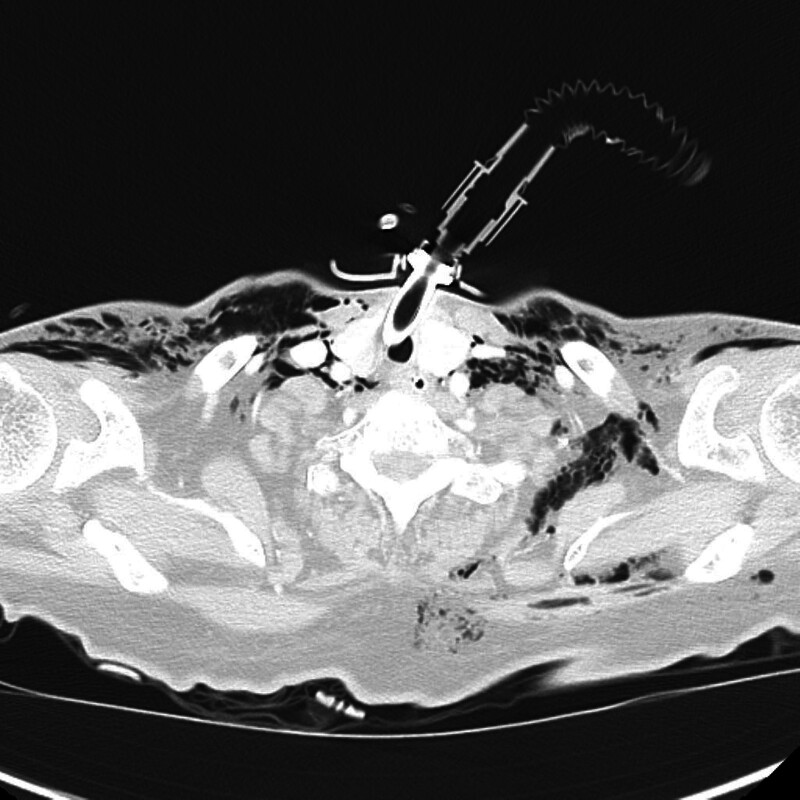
Axial chest CT image at the level of the thoracic inlet shows extensive subcutaneous emphysema. Air is noted dissecting through the fascial planes of the bilateral supraclavicular and paratracheal regions, extending into the posterior neck. A tracheostomy tube is visible in situ, and air locules surround the soft tissue structures, consistent with positive-pressure-induced barotrauma. CT = computed tomography.
